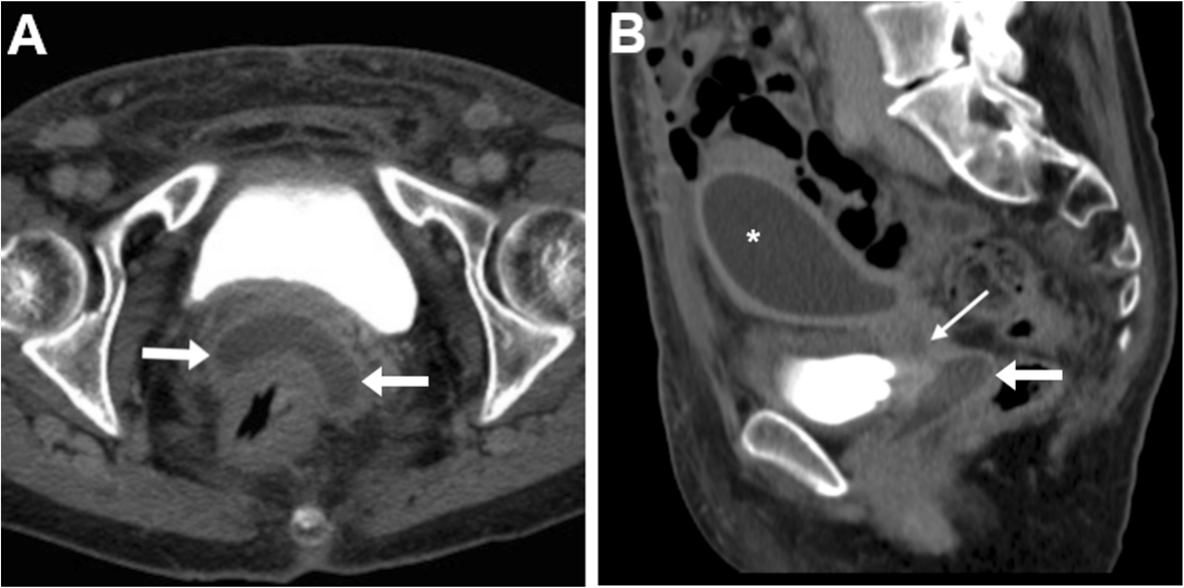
Differential diagnosis: rare case of lymphatic VF in a 67-year-old with previous laparotomic surgery for advanced ovarian cancer. CT (a, b) shows fluid-filled dilatation of the vagina (arrows) communicating via a fistula (thin arrow in b) with an ovoid fluid-attenuation pelvic collection (* in b) consistent with postoperative lymphocele
